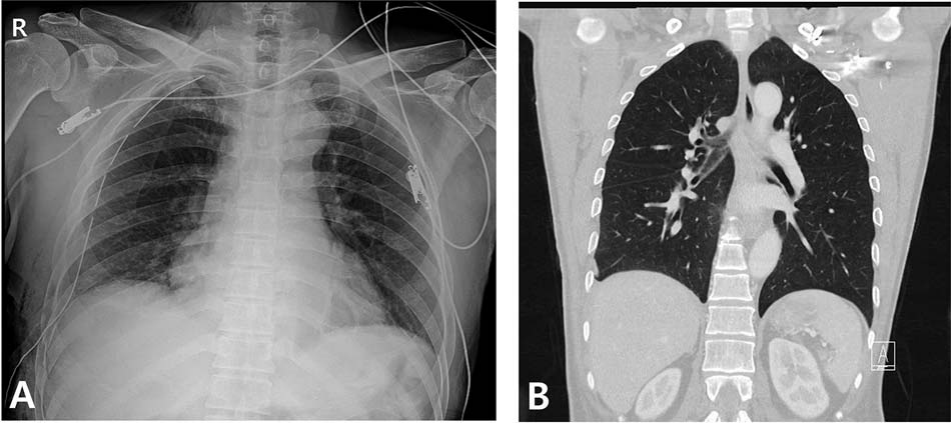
Radiography and CT scan findings. (A) Immediate postoperative chest radiography. (B) A month after surgery, outpatient chestCT.
